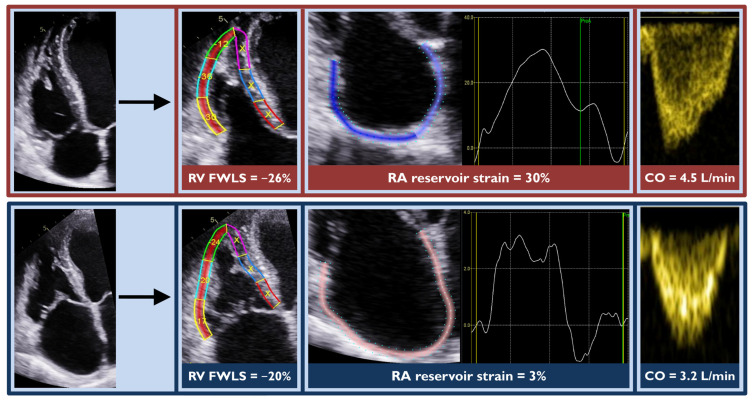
An example highlighting the main echocardiographic differences between a patient with glomerular filtration rate (eGFR) ≥ 45 mL/min/1.73 m^2^ (top row) and a patient with eGFR < 45 mL/min/1.73 m^2^ (bottom row) with acute heart failure is demonstrated. The patient with eGFR < 45 mL/min/1.73 m^2^ demonstrated more impaired right ventricular (RV) free wall strain, more impaired right atrial (RA) reservoir strain, and lower cardiac output (CO).
